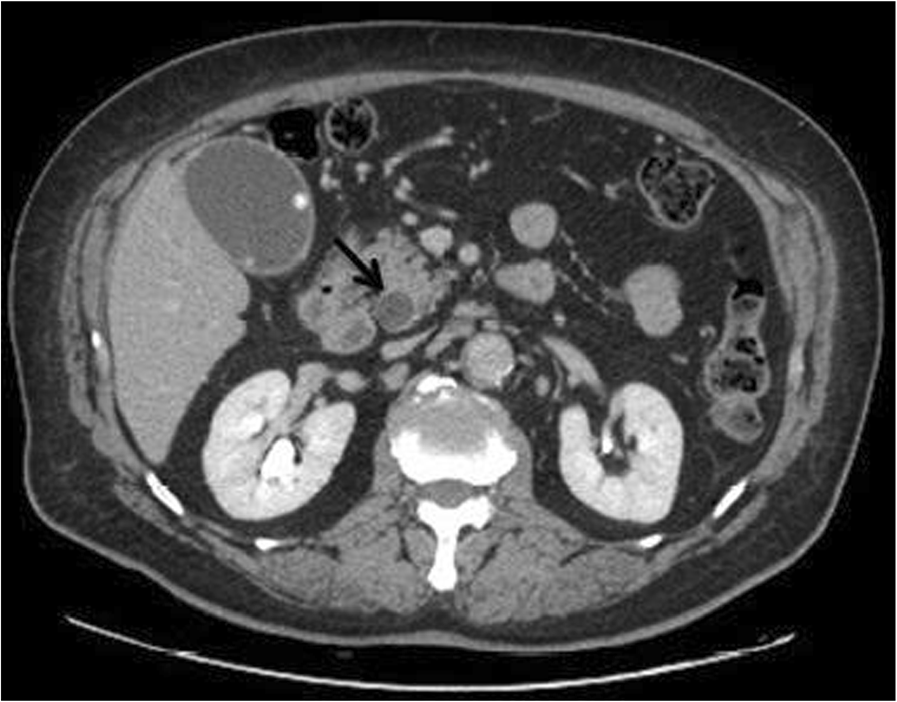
CT scan at the diagnosis. Contrast CTscan of the abdomen demonstrating an enlargement of the common bile duct (14 mm in diameter, see black arrow) and of the of the gallbladder with an inhomogeneous material into the lumen compatible with biliary sludge and microlithiasis. The extra-hepatic bile ducts swelling was evident until the upper papillary tract.
